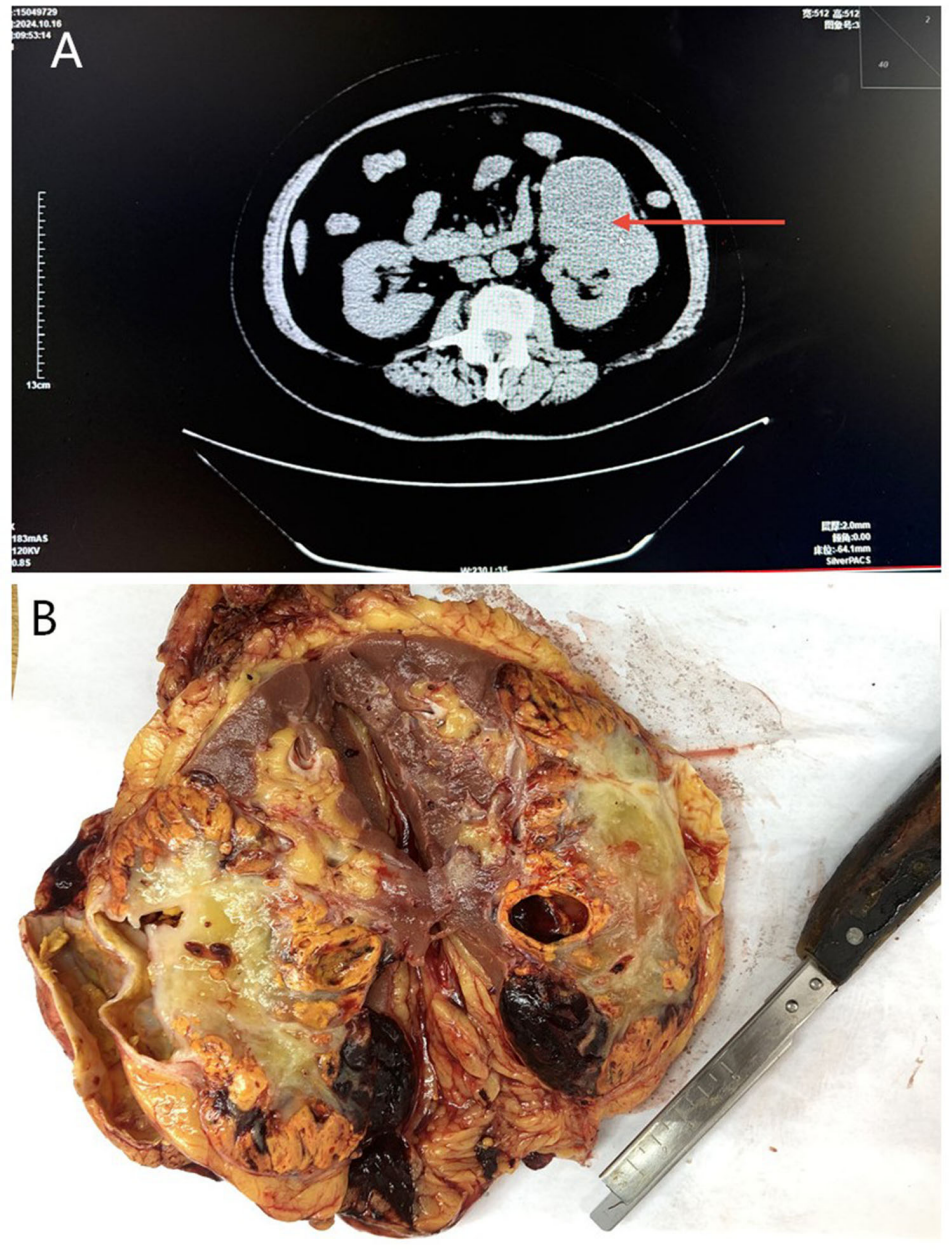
(A) Enhanced CT of the urinary system: An irregular mass measuring 11.4×9.0×7.5 cm was observed in the left renal parenchyma (red arrow), accompanied by cystic changes and calcification. The right kidney appeared normal. (B) Gross examination of the left renal tumor: The cut surface showed golden-yellow and grayish-white colors, with hemorrhage and multilocular cysts, and ill-defined boundaries.
